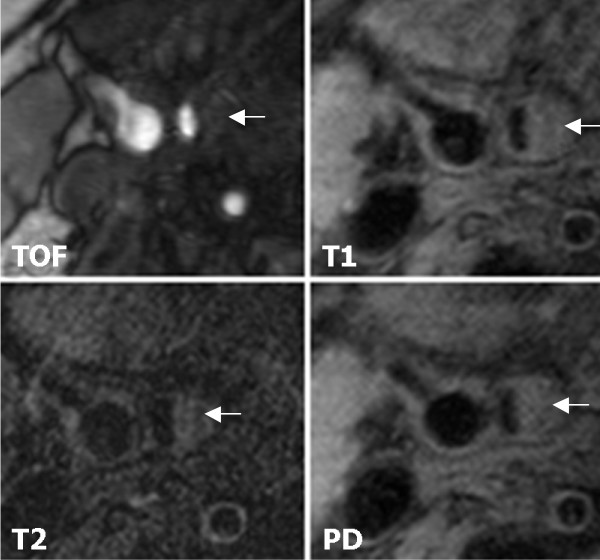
Example of lesion type VIII in the right internal carotid artery. Lesion type VIII (←) in the right internal carotid artery. MR images show iso- to slightly high-SI. Original magnification × 25.
